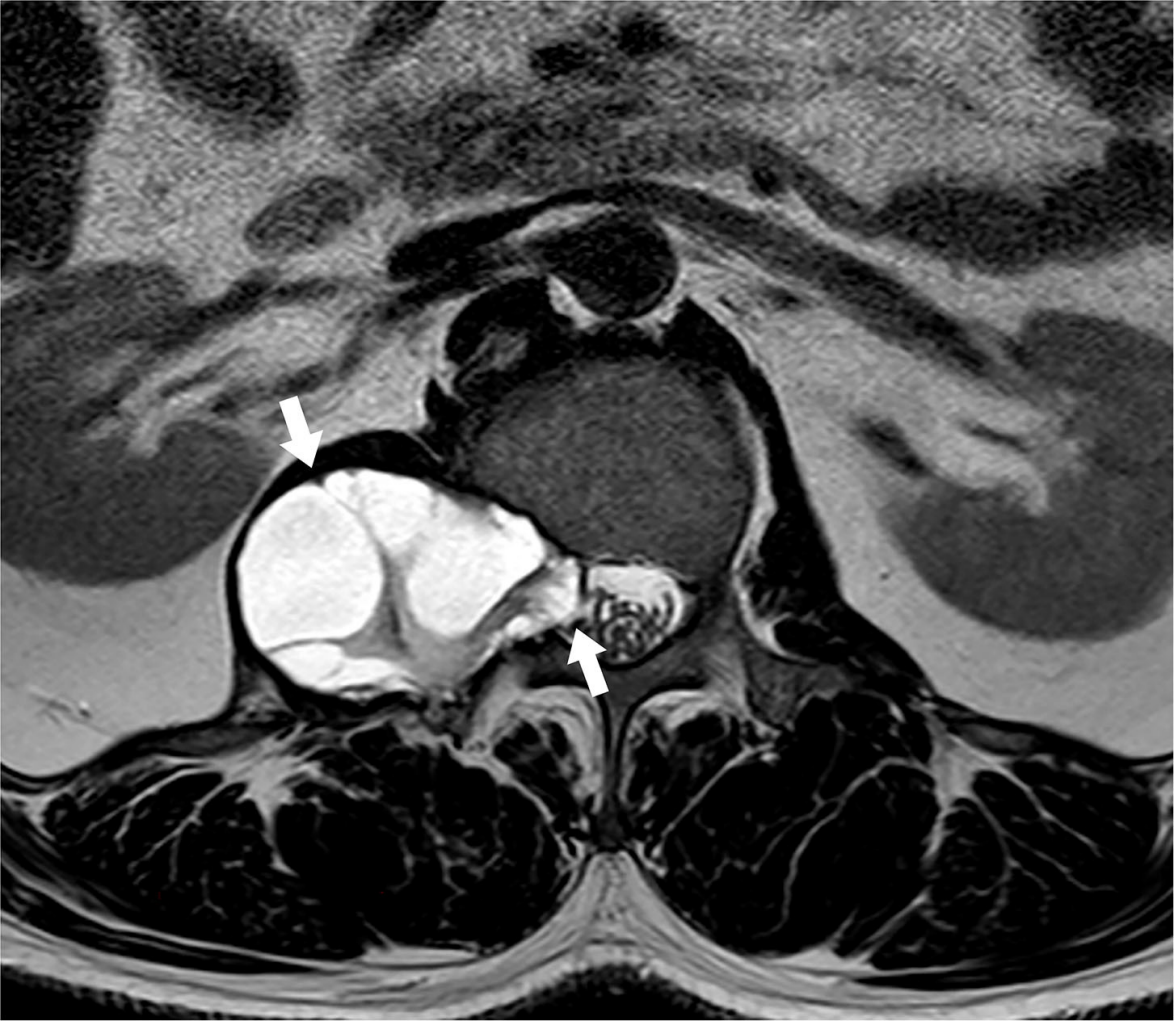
MRI T2WI (axis image) showing hyperintense signal of an oval solid-cystic cavity at the right side of the vertebral body and across through the enlarged L1~L2 intervertebral foramen. Left arrow: The part outside of the spinal canal. Right arrow: The part passing through the intervertebral foramen and inside of the spinal canal.
